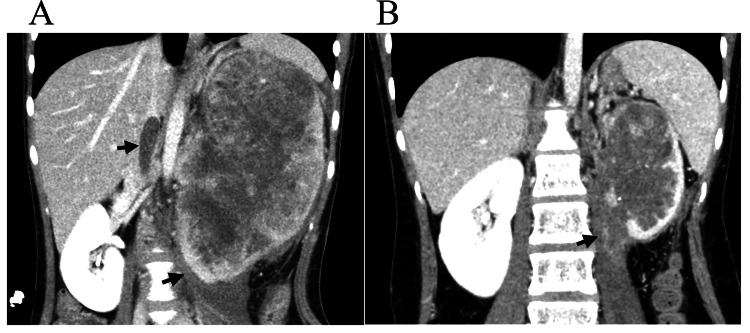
CT scan images. (A) Contrast-enhanced CT image obtained before chemotherapy demonstrating a large soft tissue mass (107 × 77 × 151 mm) originating from the left kidney, with invasion into the inferior vena cava (IVC) and left psoas major muscle. (B) Contrast-enhanced CT image obtained after chemotherapy showing a reduction in tumor size (59 × 56 × 101 mm), but psoas muscle invasion remained.
